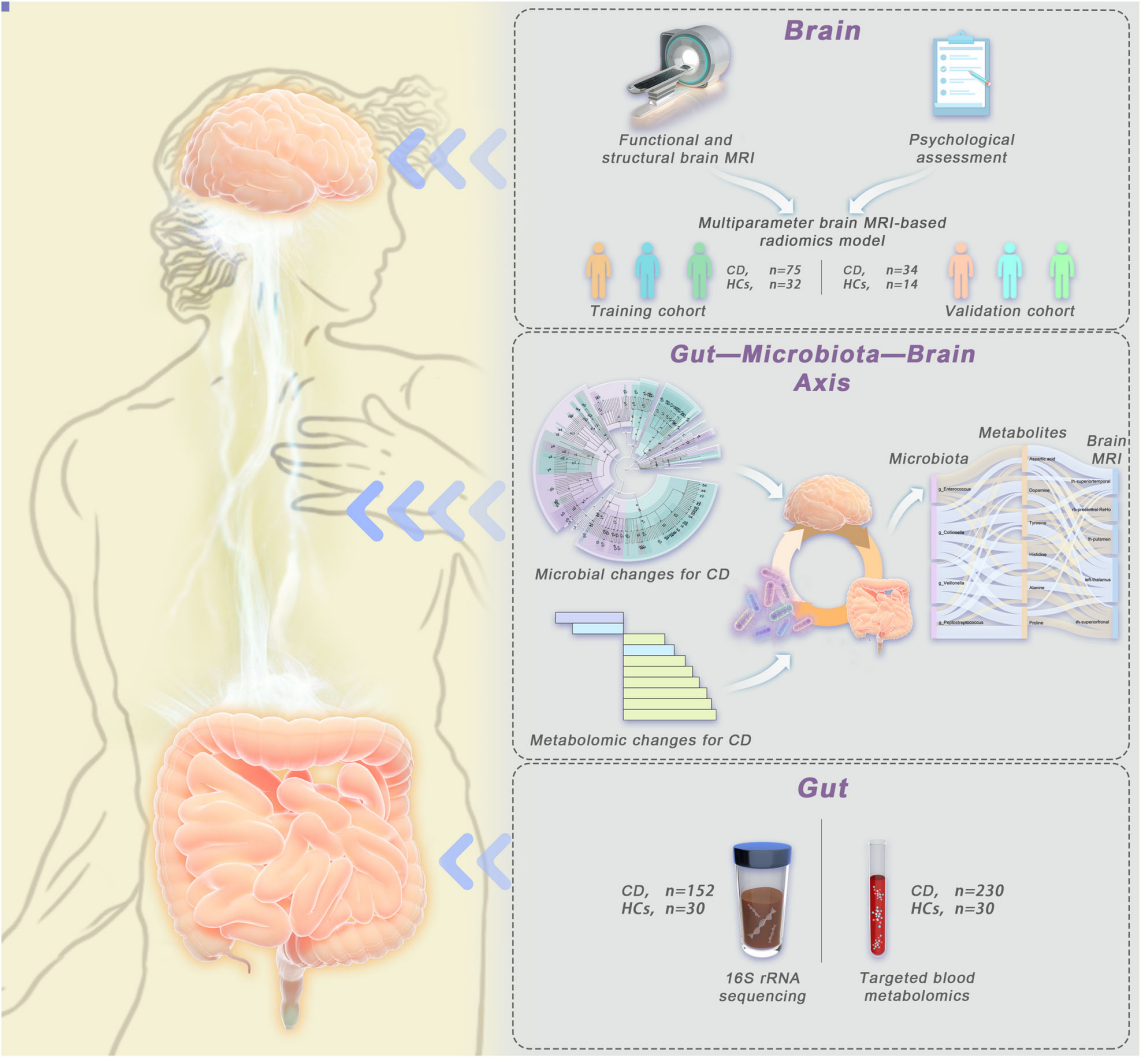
Overview of study design and analyses. We recruited a total of 276 participants (CD, *n* = 230; HCs, *n* = 46) and collected fecal samples, blood samples, multiparameter brain MRI data, and responses to a psychological questionnaire. Subsequently, a multiomics analysis of the Gut-brain axis was conducted to investigate the brain features of CD and the underlying mechanisms. (CD, Crohn’s disease; HCs, healthy controls; 16Sr RNA, 16S ribosomal ribonucleic acid)
